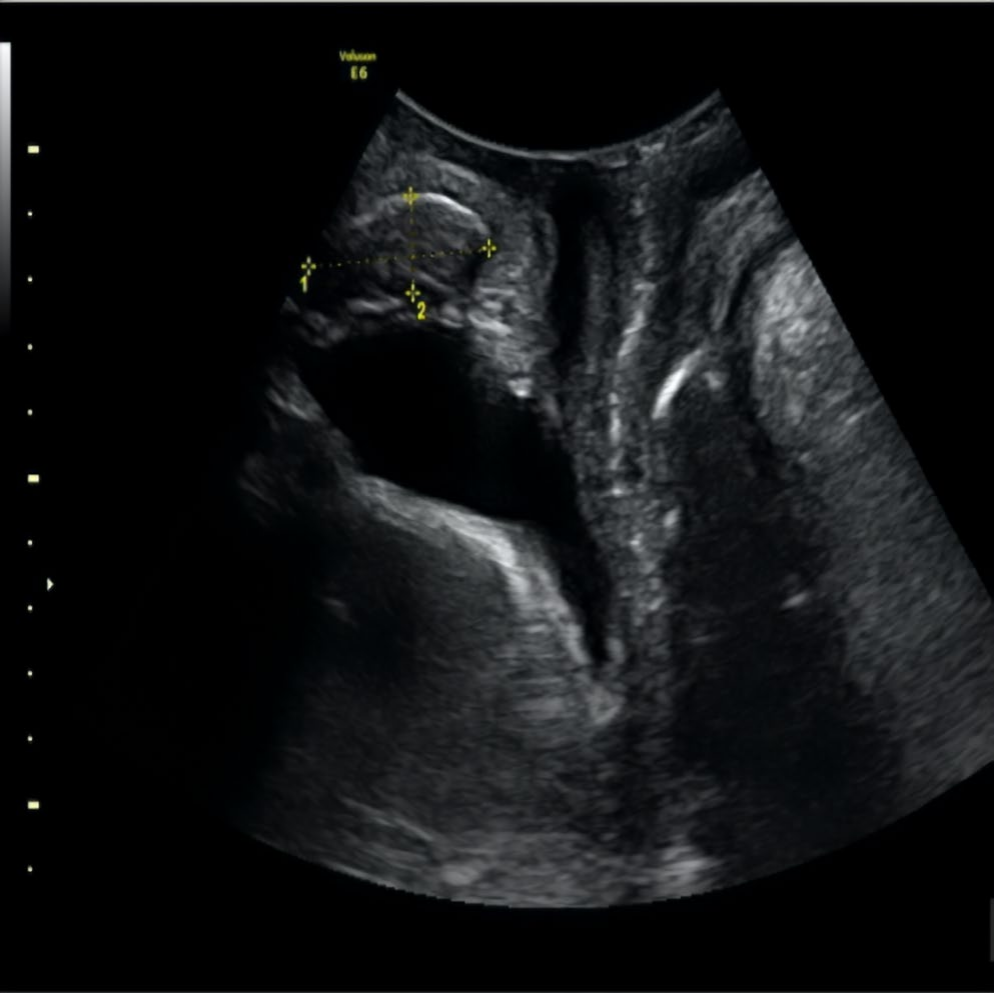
Translabial ultrasonic image; a lesion of 36 × 15 mm is being present in the median tissue between the abdominal wall and the subcutaneous tissue
